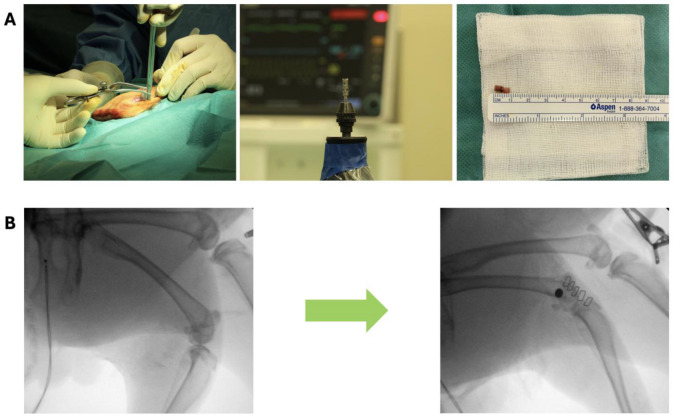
(A) Surgical approach and preparation followed by drilling of the bony defect of 4.5 × 8 mm; (B) pre- and post-operative X-ray.
